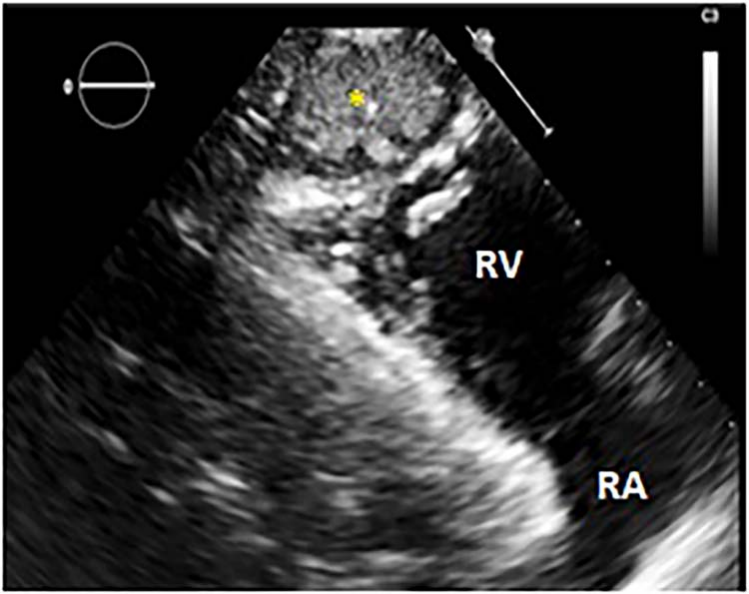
TTE: modified parasternal long axis view showing a hyperechoic cystic mass (yellow star) next to the right ventricular apex. RV: right ventricle; RA: right atrium.
